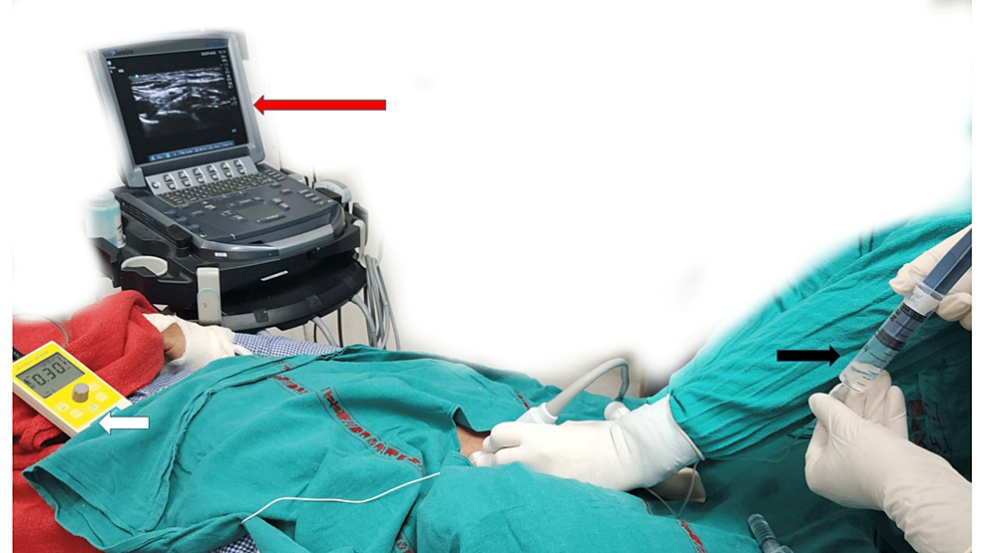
Multimodal approach for nerve localization and block administration. Red arrow: ultrasound for nerve localization, white arrow: nerve stimulator, and black arrow: compressed air injection technique.
